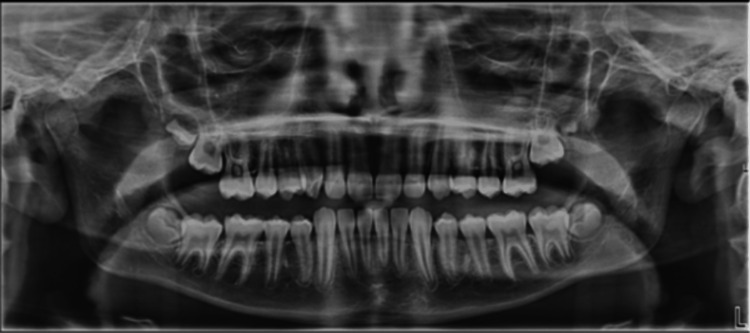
Initial orthopantomogram confirming no dental pathology
